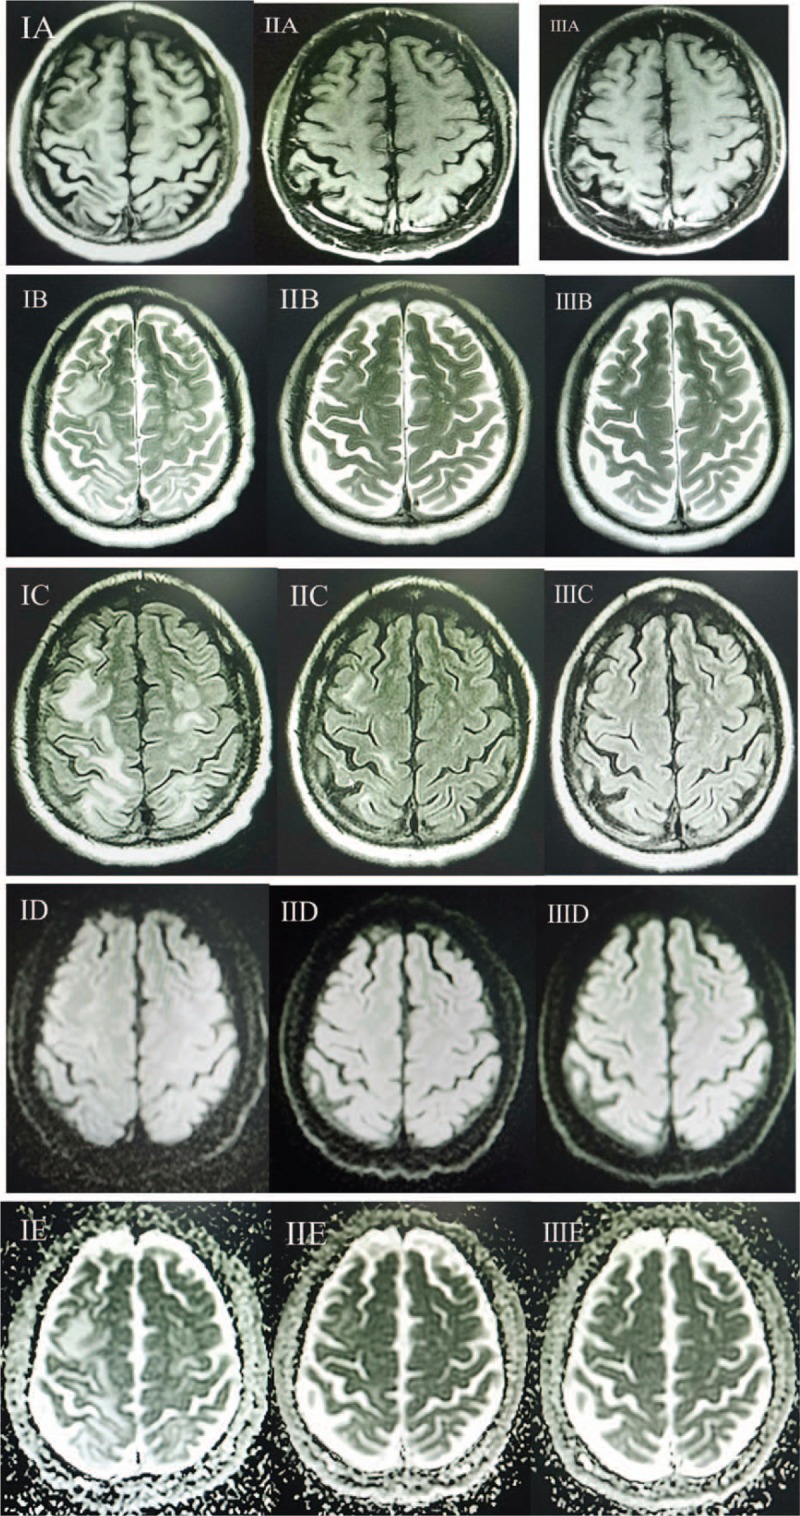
Brain magnetic resonance imaging (MRI). I, Brain MRI scan on August 2, 2018. II, Brain MRI scan on August 13, 2018. III, Brain MRI scan on September 5, 2018, respectively. A, MRI T1 FLAIR, B, MRI T2, C, MRI T2 FLAIR, D, DWI, E, ADC, respectively. ADC = apparent diffusion coefficient, DWI = diffusion-weighted imaging, FLAIR = fluid-attenuated inversion recovery.
